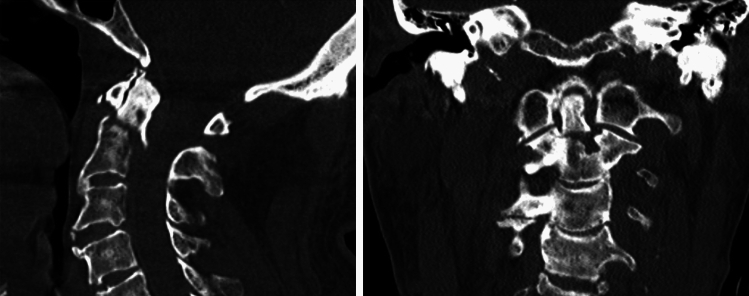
Sagittal and coronary CT view of an 89-year-old male who suffered OHCA in relation to a fresh Odontoid “Anderson II” fracture after a ground level fall. Initial cardiac rhythm was PEA. ROSC was reached after a total of 25 min of CPR (layman and professional CPR combined) and the admission of 1 mg of intravenous adrenaline. Exitus letalis occurred within 24 h in the intensive care unit
